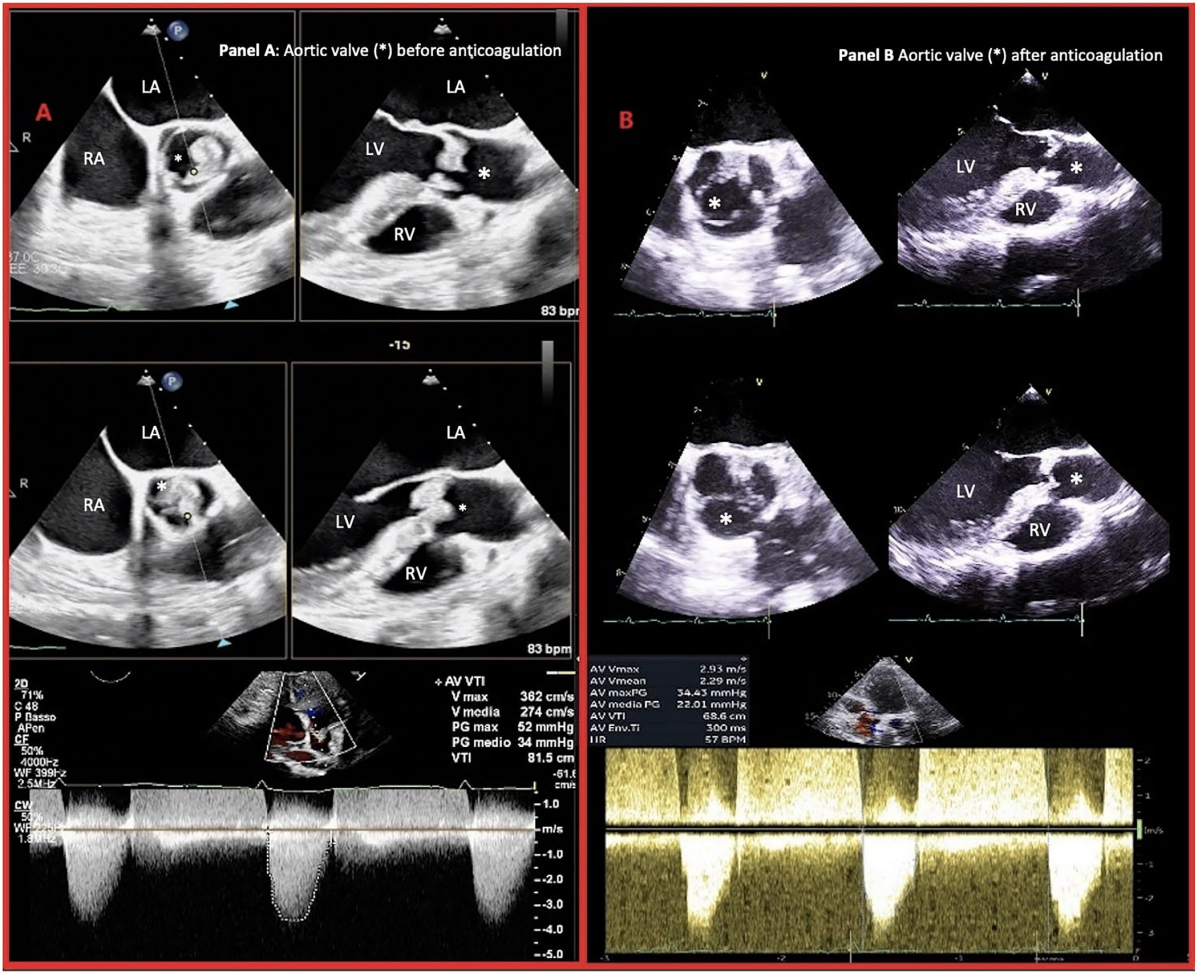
Transoesophageal echocardiography performed before (Panel A) and after (Panel B) anticoagulation.
